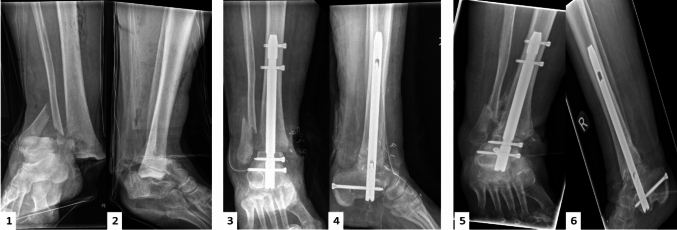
Isolated open ankle fracture dislocation in a female 85 years of age sustained after a fall from standing height with poor soft tissue conditions. (1, 2) preoperative AP and lateral radiographs of the ankle; (3, 4) immediate postoperative AP and lateral radiographs of the ankle demonstrating stabilization of the ankle and subtalar joint with the Phoenix Ankle Arthrodesis Nail; (5, 6) AP and lateral ankle radiographs showing ankle and subtalar fusion 8 months postoperatively
